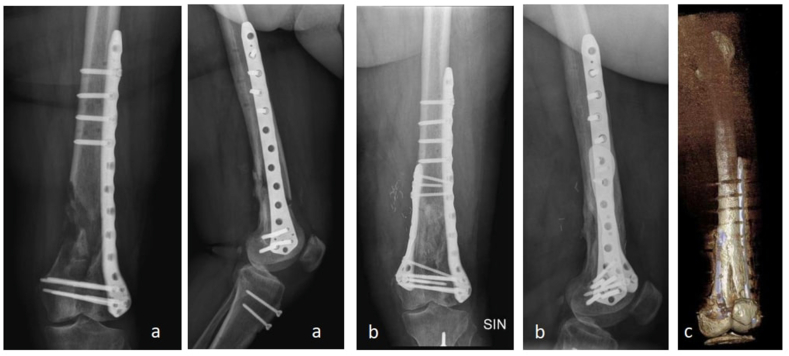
Clinical case n°1. Pre-op X-rays (a), post op X-rays (b) and 3D CT scan (c) results at 12 months.
